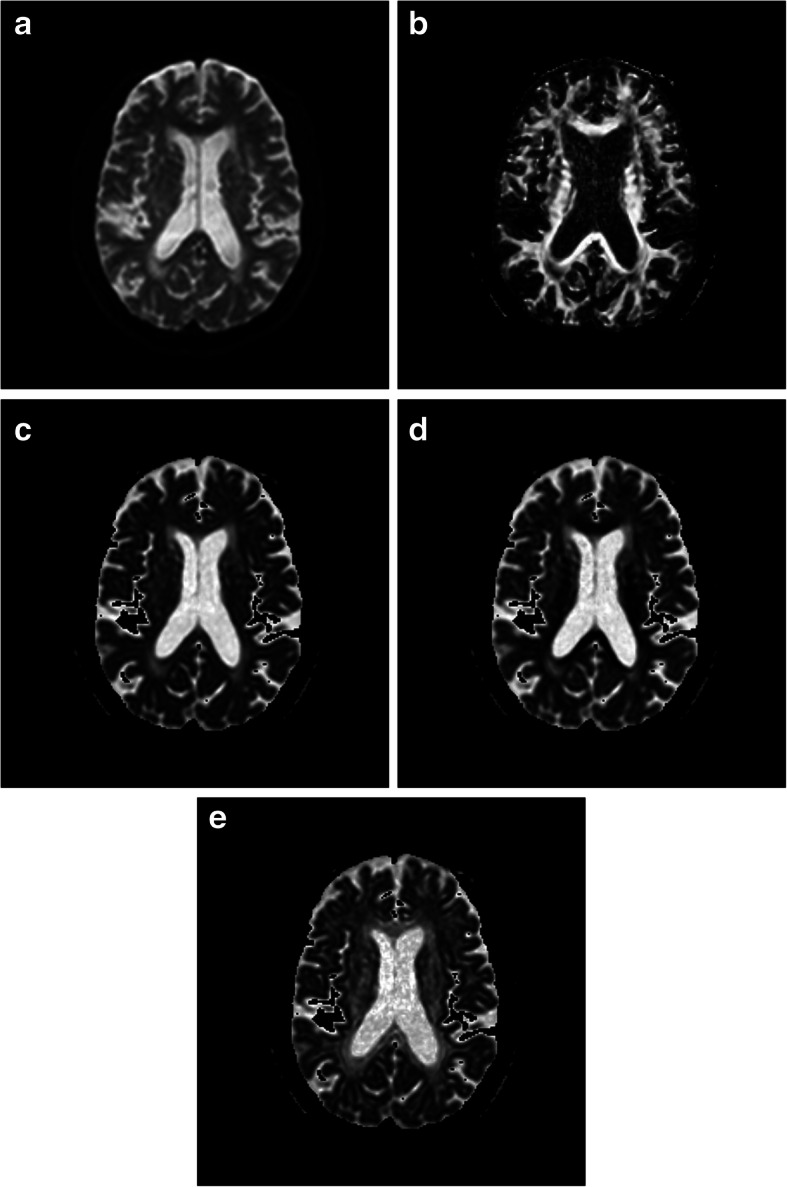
The b0 of the DWI (a) of a 77 years old male showed WMH (Fazekas scale 2) in the pFL, pOH, pPL and dCO. FA (b) of WMH in the pFL are lower than that of pOL, pPL and dCO. MD (c) and RD (d) of WMH in the pFL are higher than that of pOL, pPL and dPCO. AD (e) of WMH in the pFL are close to that of pOL, pPL and dCO
